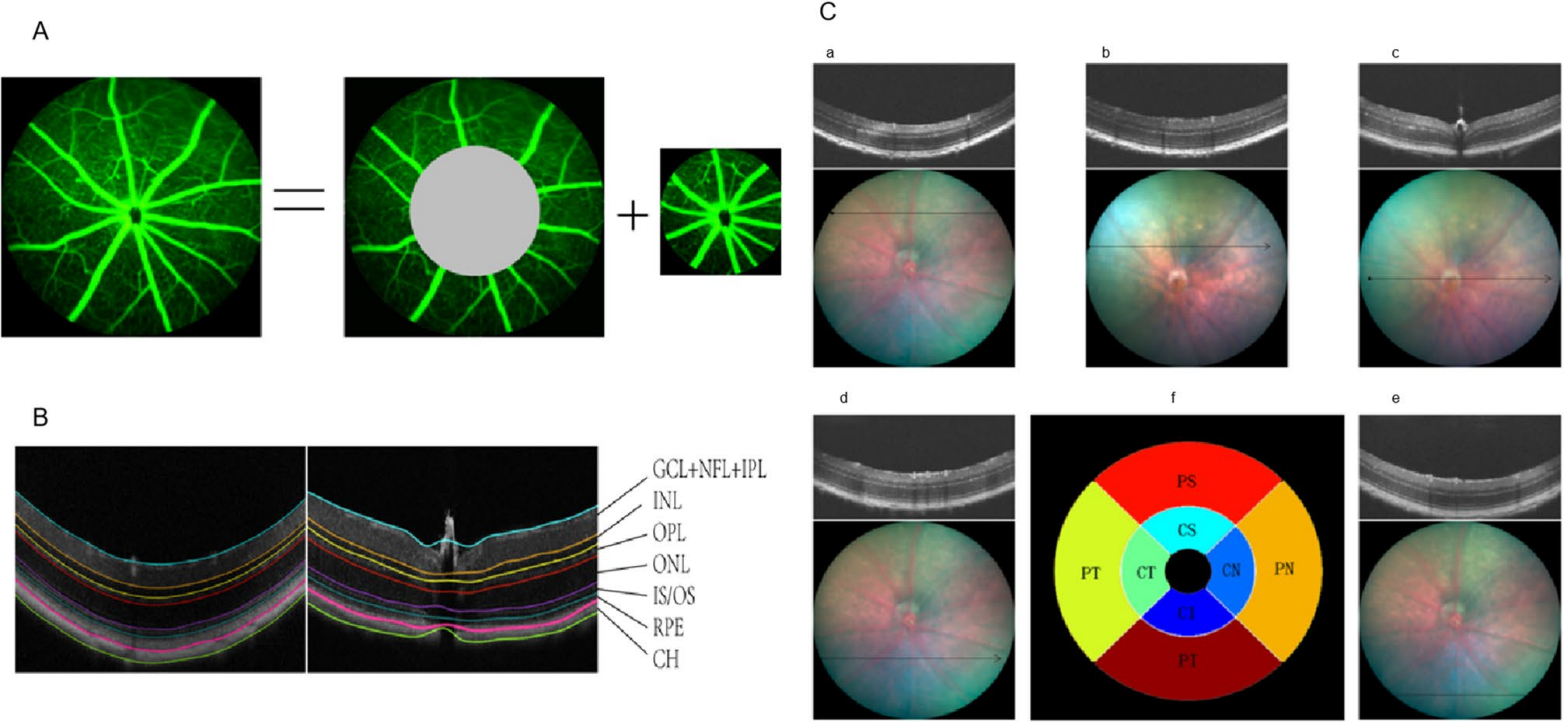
Retinal fundus fluorescein angiography and fundus photography corresponding with the cross-sectional OCT images. A FFA images include central and peripheral region. The central region is defined as three times the diameter of the optic disc, the rest of the retina considered as the peripheral region. B Seven targeted retinal layer boundaries in SD-OCT scan. C The upper (a), upper-middle (b), middle (c), middle-lower (d), and lower areas (e) of the retina were scanned. The scanning lines positioned 3 PD and 1.5PD from the optic disc separately. When measuring the central area, a full-length retinal scan across the middle of disc (e); The reproducibility measures were computed in the eight regions shown above, namely, the CS, CN, CI, CT, PS, PN, PI, and PT regions. The inner, middle, and outer circles are 0.2, 0.6, and 1.2 mm in diameter, respectively (f).
